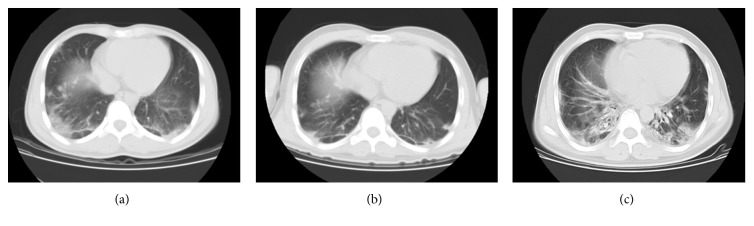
CT scan of patient in different stages. (a) A chest CT scan showing exudative lesions when he came to our hospital for the first time. (b) After treatment, the ILD gradually improved. (c) A CT scan indicating advanced ILD when the patient came to our hospital again.
